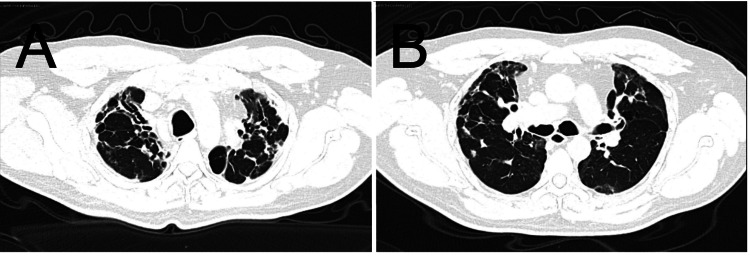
Chest CT findings. (A) Axial chest CT demonstrating residual fibrotic changes in the upper lobes consistent with prior granulomatous infection.
